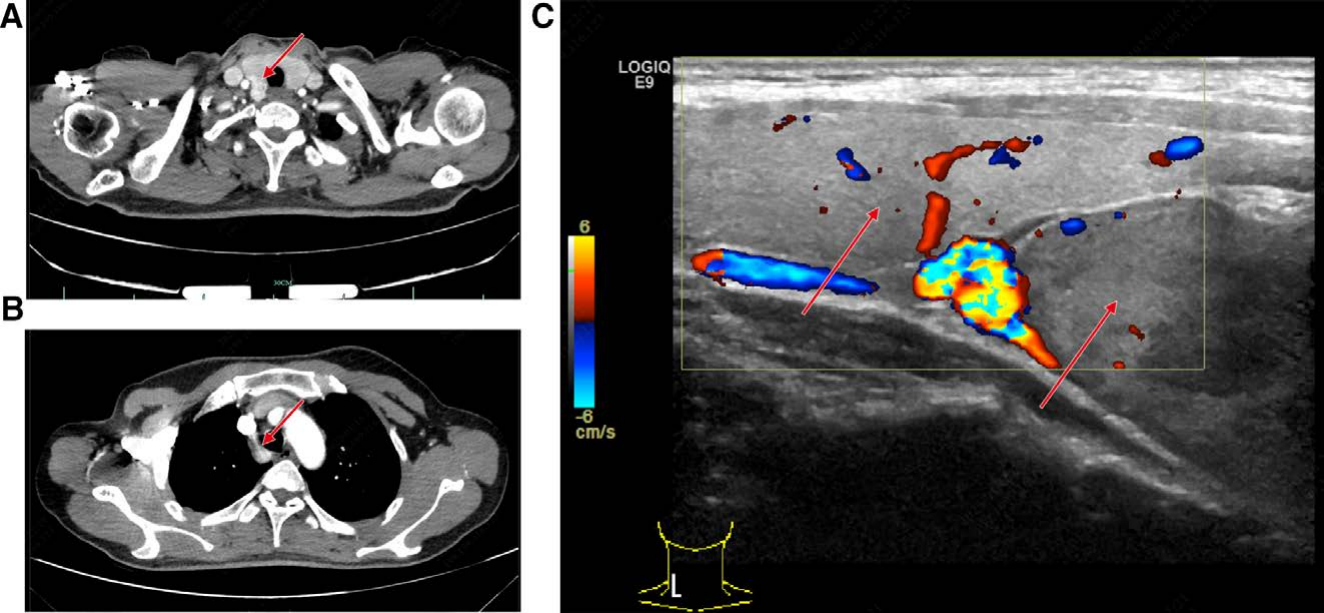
(A) CT scan images of the right anterior superior mediastinal parathyroid adenoma. The arrow indicates the upper edge of the parathyroid adenoma located behind the right thyroid gland. (B) CT scan images show the lower edge of the right anterior superior mediastinal parathyroid adenoma at the level of the aortic arch, as indicated by the arrow. (C) Ultrasound examination images of the parathyroid glands in the neck. The left arrow points to the right thyroid gland, and the right arrow points to the right parathyroid adenoma, located near the lower edge of the thyroid gland.
